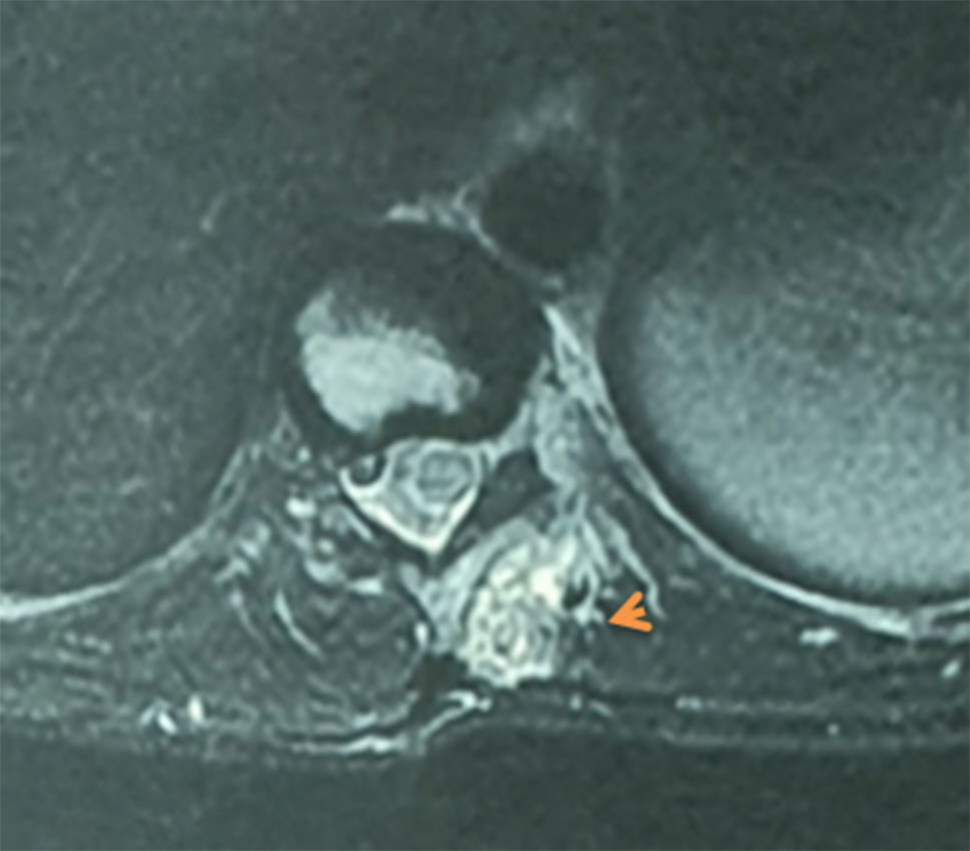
MRI showed signal abnormalities in the left superior articular process of T11, an intense response of peripheral soft tissues
